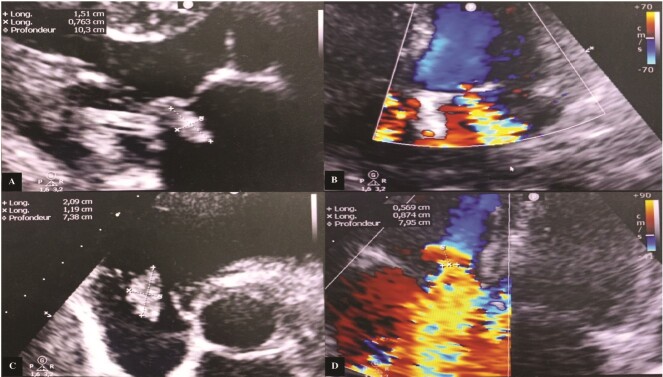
Transthoracic echocardiography images. (A) Vegetation attached to the atrial side of the anterior mitral leaflet measuring 15 × 7.6 mm. (B) Significant mitral regurgitation. (C) Vegetation attached to the free edge of the anterior tricuspid leaflet measuring 21 × 12 mm. (D) Massive tricuspid regurgitation.
